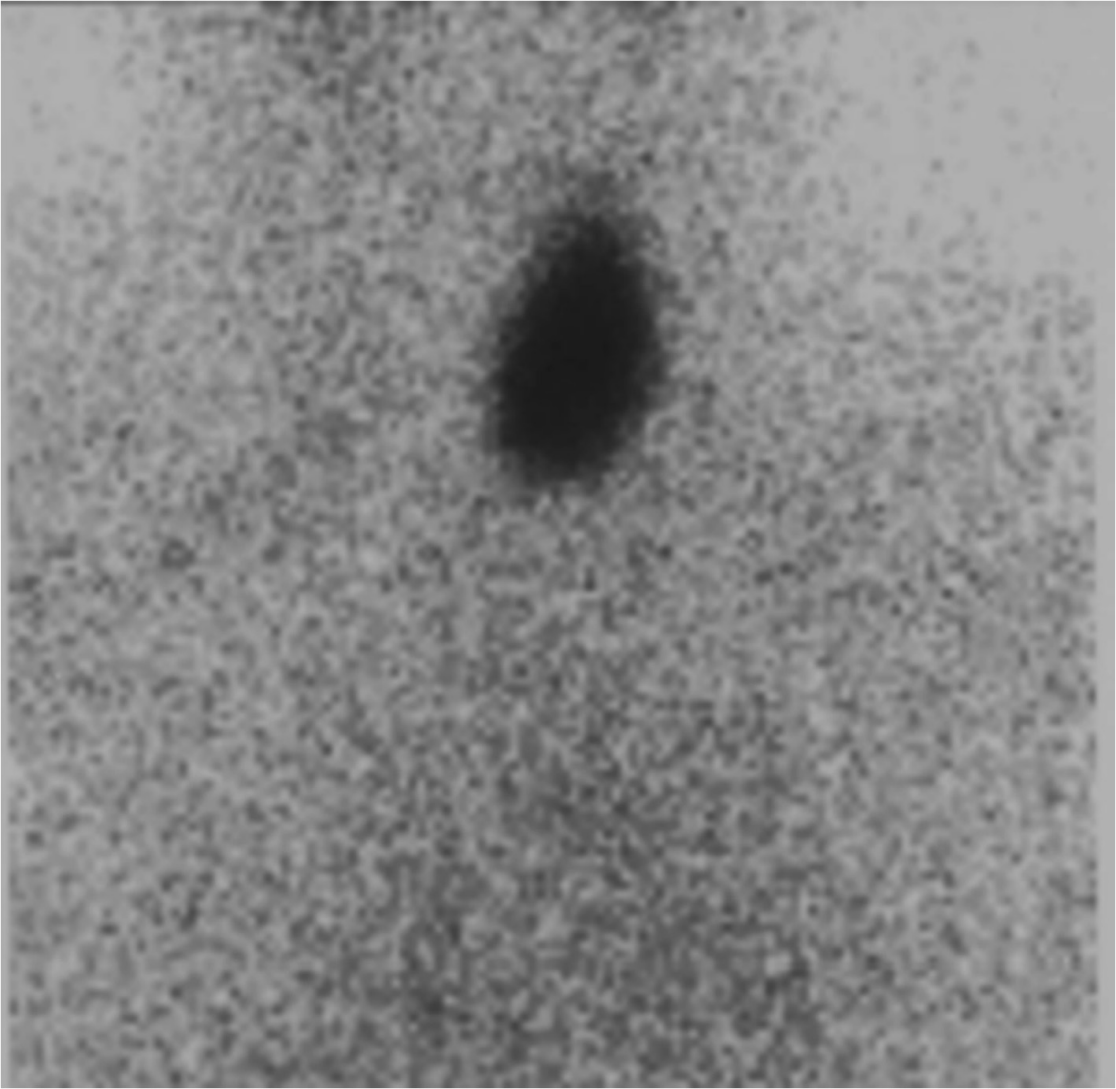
Neck scintigraphy (MIBI).
